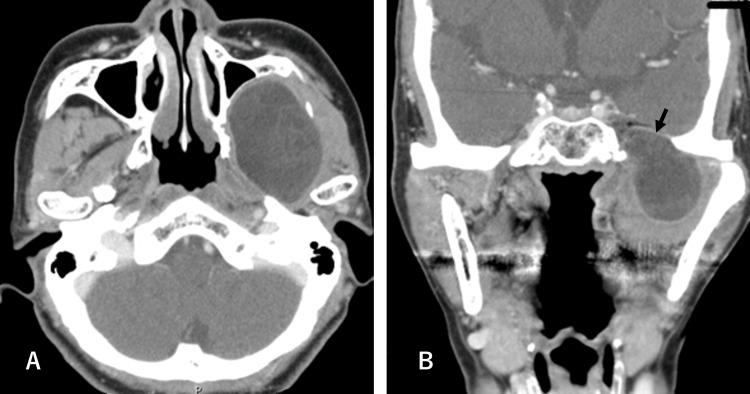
Preoperative computed tomography (CT) scans of Case 2. (A) Axial and (B) coronal views reveal a well-defined cystic lesion in the left infratemporal fossa. Thinning of the skull base bone is observed (arrow in B), likely due to the expansion of the cyst wall.
